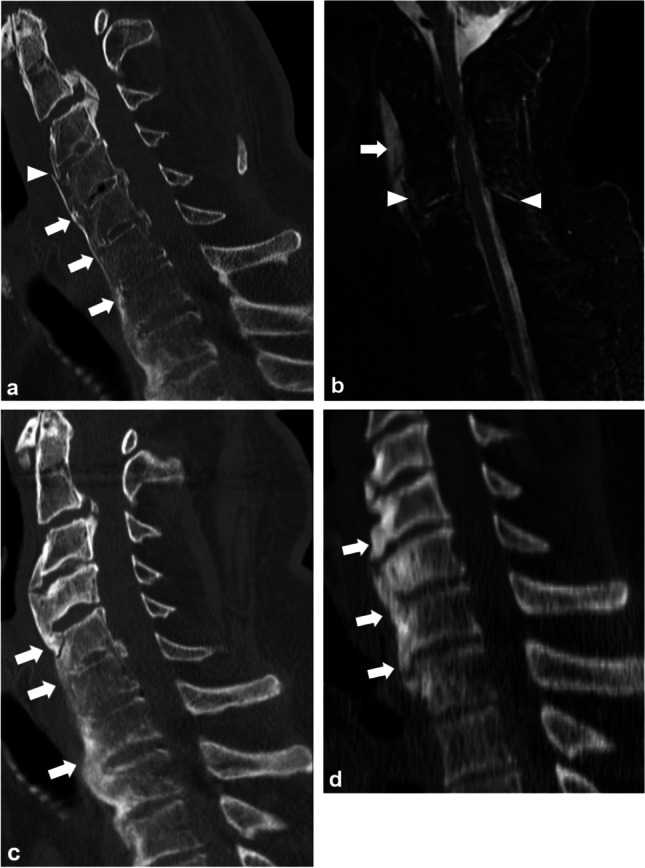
A 60-year-old male after GLF to his lower back without direct impact to the head or the neck. A sagittal CT image shows anteriorly bridging syndesmophytes (arrows) with features of both ankylosing spondylitis and DISH. A small fracture (arrowhead) is evident at the calcified anterior longitudinal ligament of the intervertebral space between the third and the fourth cervical vertebra (a). The fracture (arrowheads) running through the anterior body of the fourth cervical vertebra and along the upper endplate of the fifth cervical vertebra continuing to the ligamentum flava and the interspinous ligament is more distinct on sagittal STIR, which also reveals a prevertebral hematoma (arrow) (b). A sagittal CT image taken 6 years prior to injury shows more prominent bridging syndesmophytes (arrows) at the anterior parts of the intervertebral discs (c). A sagittal CT reformat from lower-resolution images taken 16 years prior to injury shows recognizable syndesmophytes (arrows) indicative of DISH (d). MRI = magnetic resonance imaging, STIR = short tau inversion recovery, GLF = ground level fall
